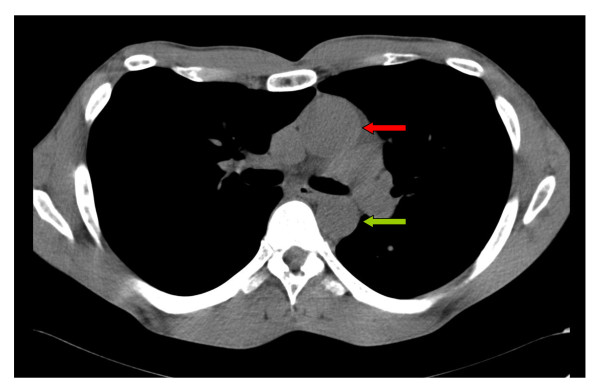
Initial CT-scan showing the ascending (red arrow) and descending aorta (green arrow). The ascending aorta appears to be significantly more dilated as it should be.
